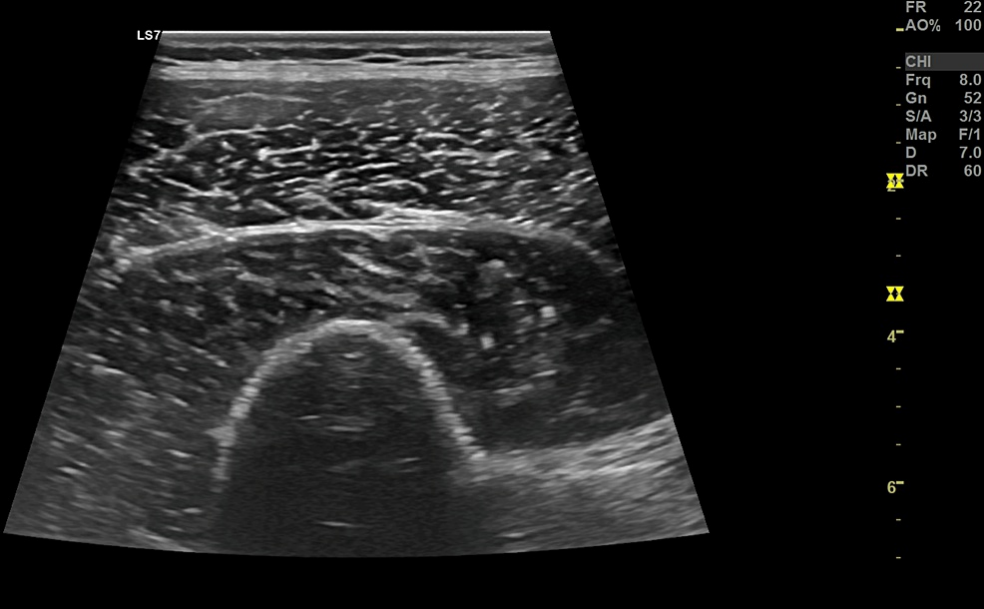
Complementary study: Soft-tissue ultrasound of the left thigh performed by Radiology No expansive lesions were observed in this topography, maintaining the normal fibrillar structure of the muscles. There was no fluid collection.
